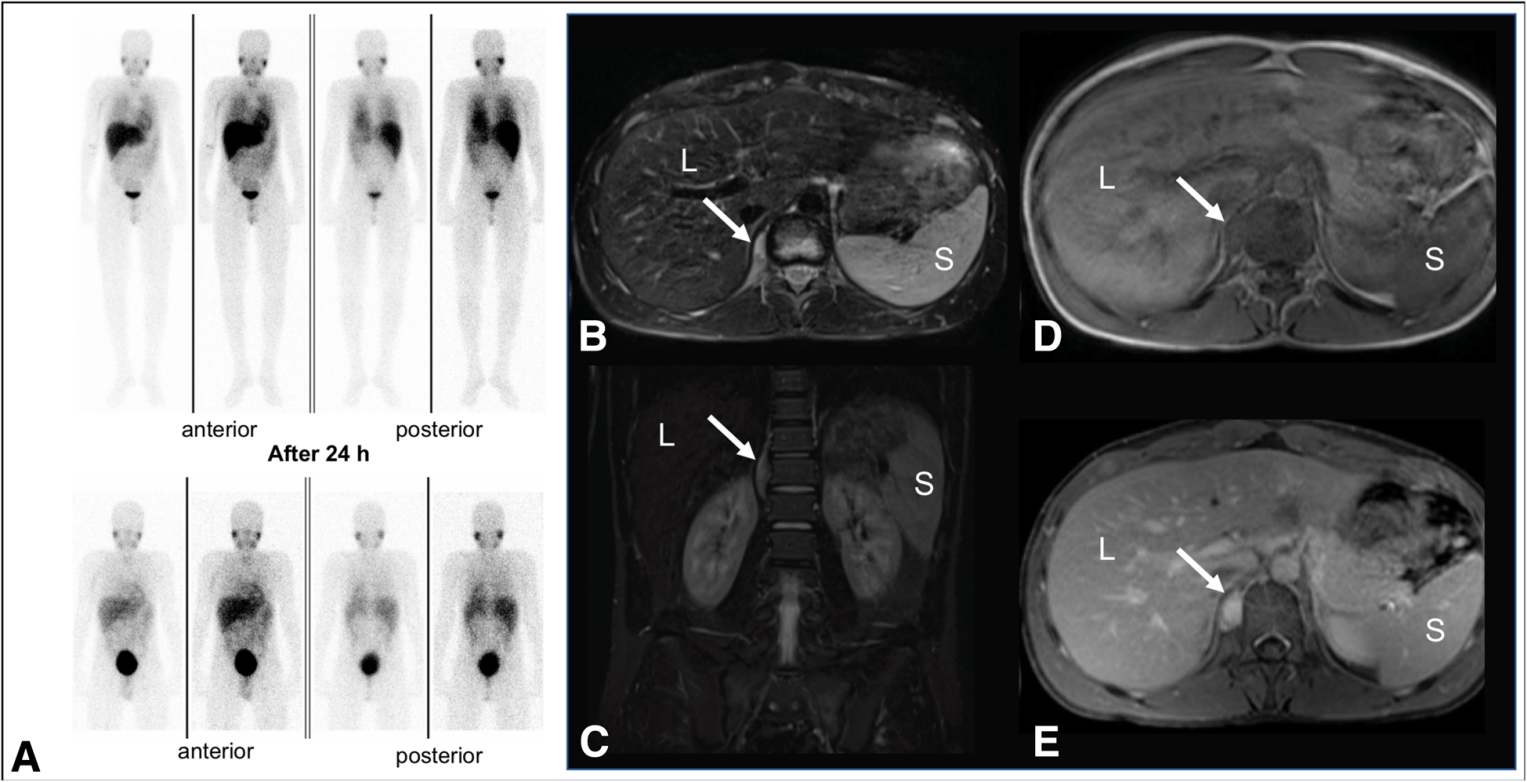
Case 3: 13 year old boy presenting with steroidresponsible opsoclonus-myoclonus syndrome. No I-123 MIBG tracer uptake could be detected in scintigraphy after the administration of 108 mBq I-123-mIBG (a). WB-MRI revealed a paravertebral right-sided retroperitoneal mass (*white arrow*) at the level of T11 to L1 with hyperintensity in T2 weighted sequences (b, axial T2w and c, coronal) and contrast enhancement in the post-contrast T1 weighted sequence after intravenous injection of 7 ml gadolinium compound (d, native T1 weighted sequence and E, post-contrast T1 weighted sequence). *L = liver, S = spleen*
